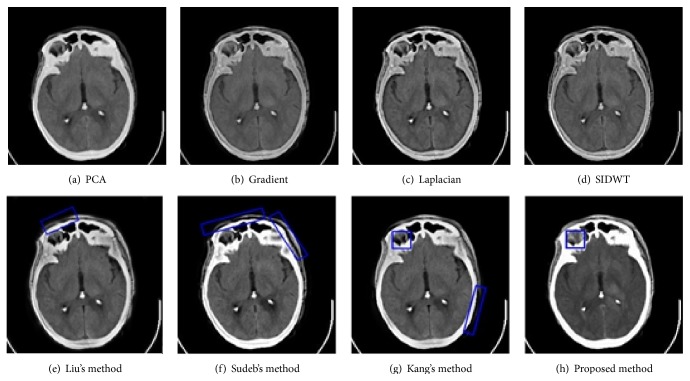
The fusion results of CT and MRI.
